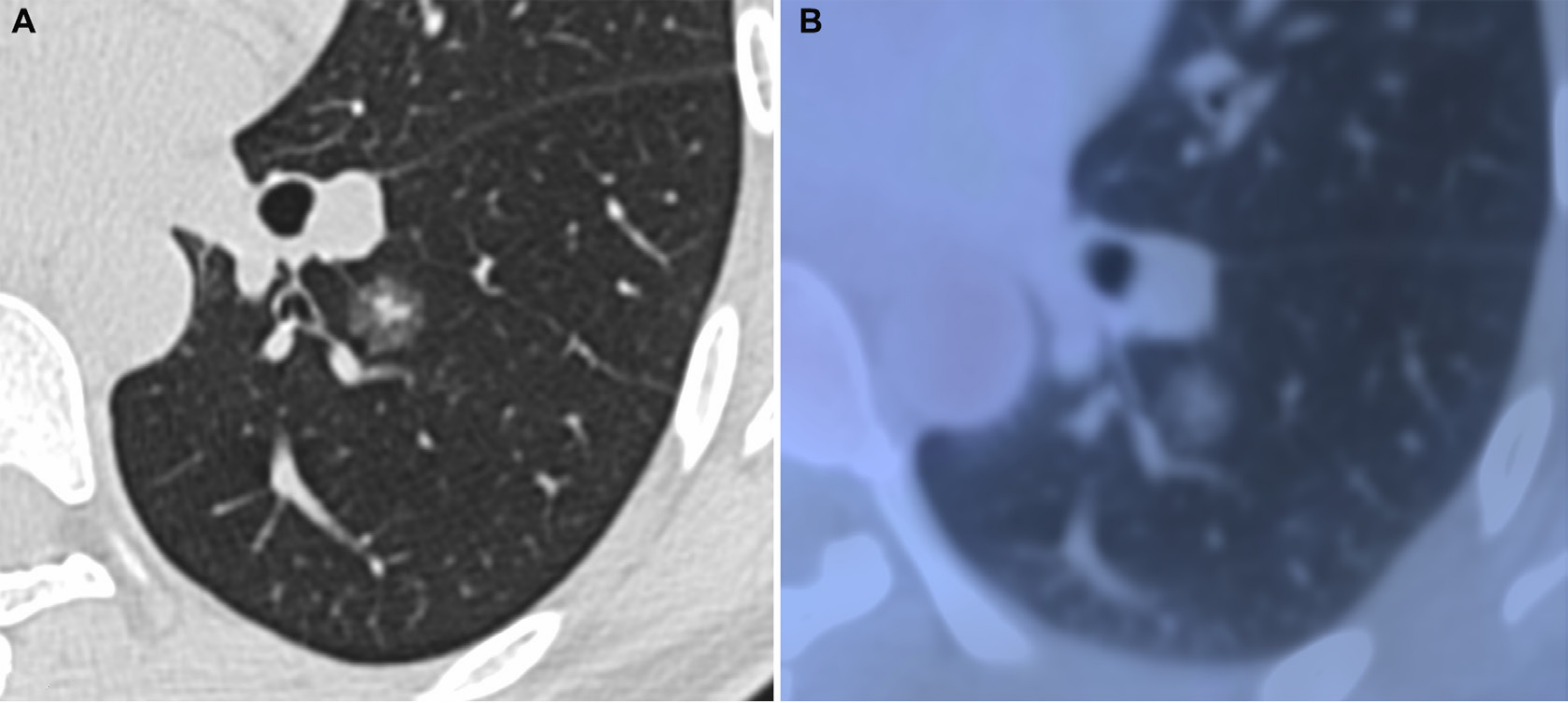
Minimally invasive adenocarcinoma in 40-year-old woman (A) transverse lung-window HRCT scan demonstrates a round, spiculated, well-defined parenchymal mixed GGN with pleural indentation in the apical segment of the left lower lobe. (B) PET/CT fusion image with perfusion mode shows a 11.9-mm mixed GGN with 0.25 of CTR and 0.81 of SUV_max_.
